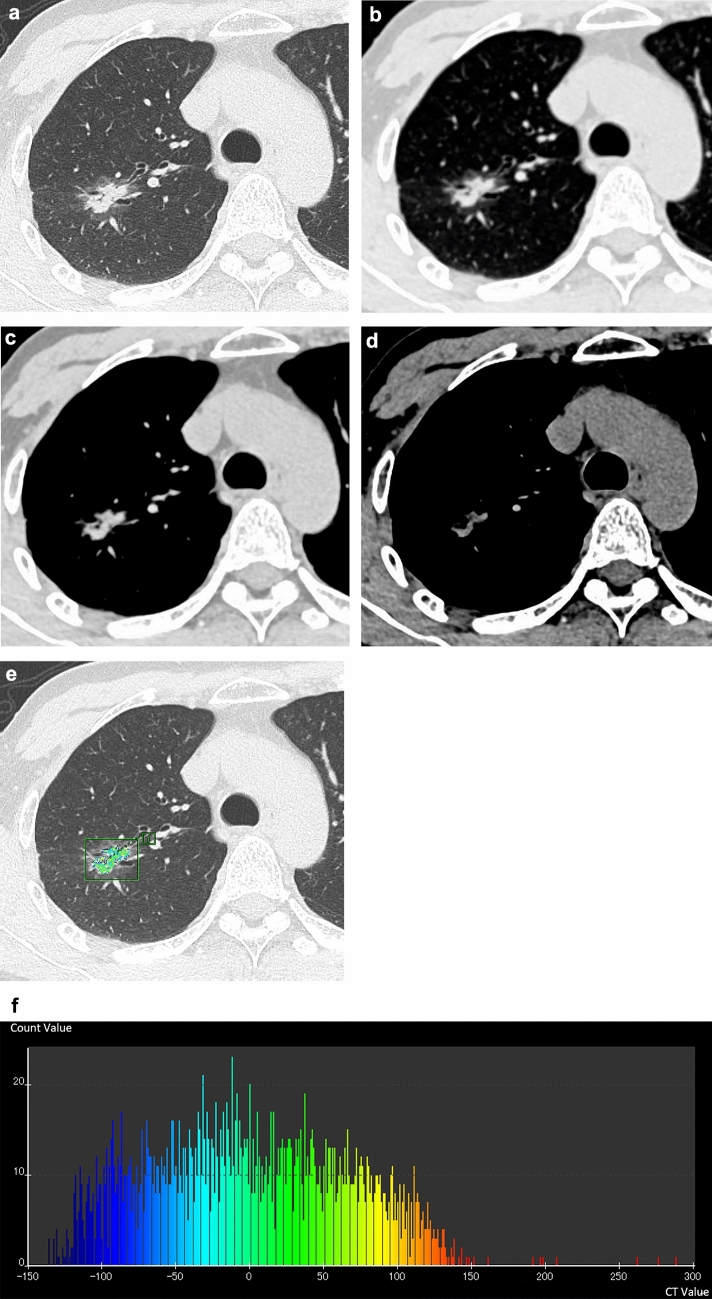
(A–D) A 59-year-old man with invasive adenocarcinoma. HRCT shows a part-solid nodule and the solid component is also observed in the mediastinal window setting. Diameter of the entire tumor = 2.7 cm, solid component = 2.06 cm (A), 1.93 cm (B), 1.91 cm (C), and 1.58 cm (D). The mean CT value of the solid component was 45.5 HU. (E, F) The volumes, and the 50 th, 70 th, and 100 th percentiles of the solid component were 0.9 cm^3^, −4 HU, 51 HU, and 288 HU, respectively
